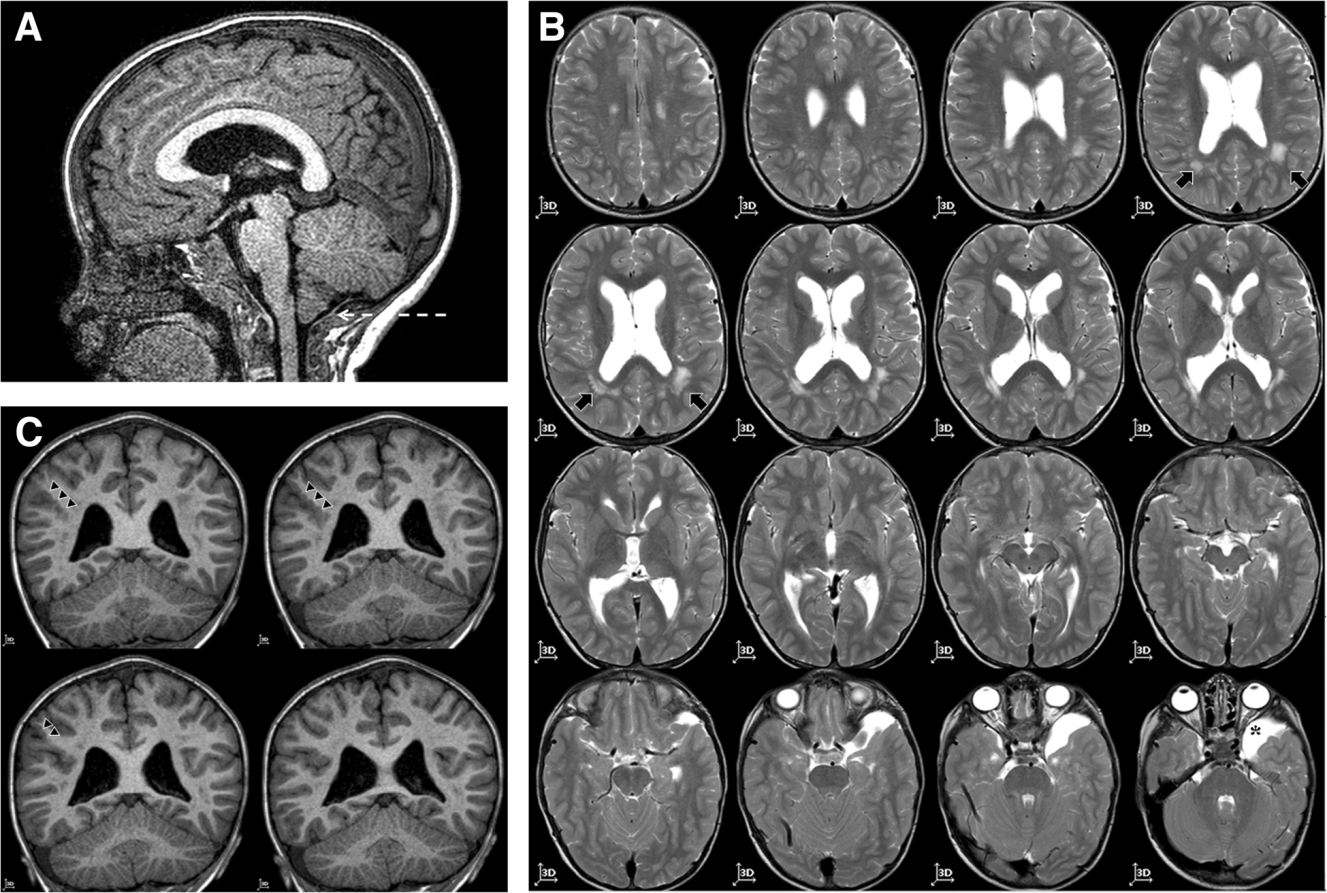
Brain MRI: markedly thick corpus callosum and Chiari malformation type I (highlighted by the white arrow) represented in panel a; abnormalities of white matter (highlighted by the black arrow) and arachnoid left temporo-polar cyst (highlighted by the asterisk) represented in panel b; area of polymicrogyria in right parietal region (highlighted by the black triangles) represented in panel c
